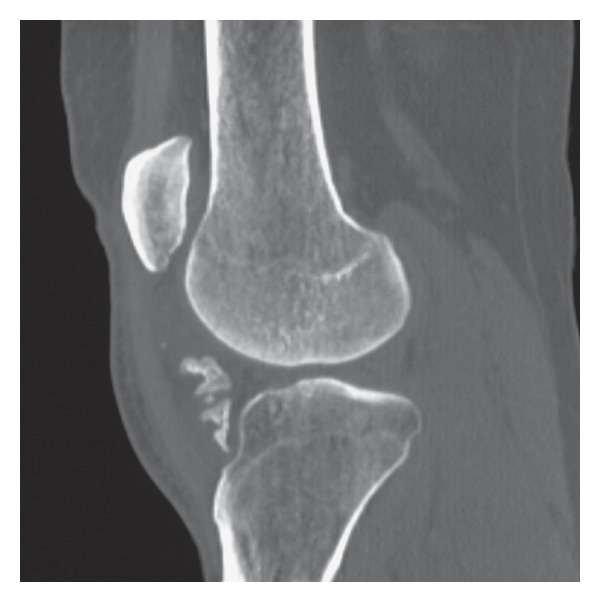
Computed tomography showing the mass with its osseous content in the infrapatellar region.
